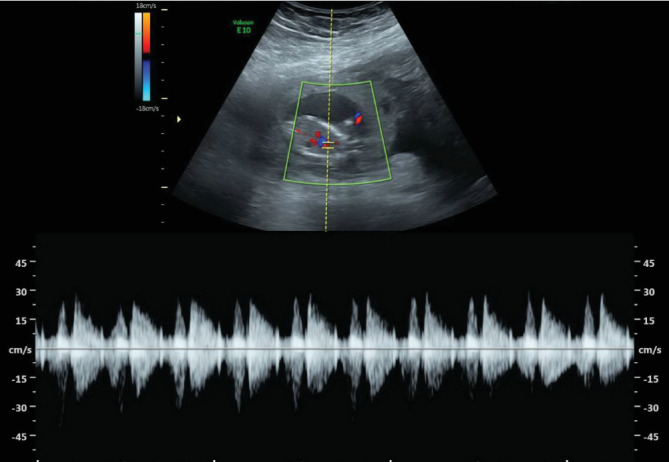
Ultrasound shows extrauterine pregnancy and the fetal heart rate (176 bpm).
